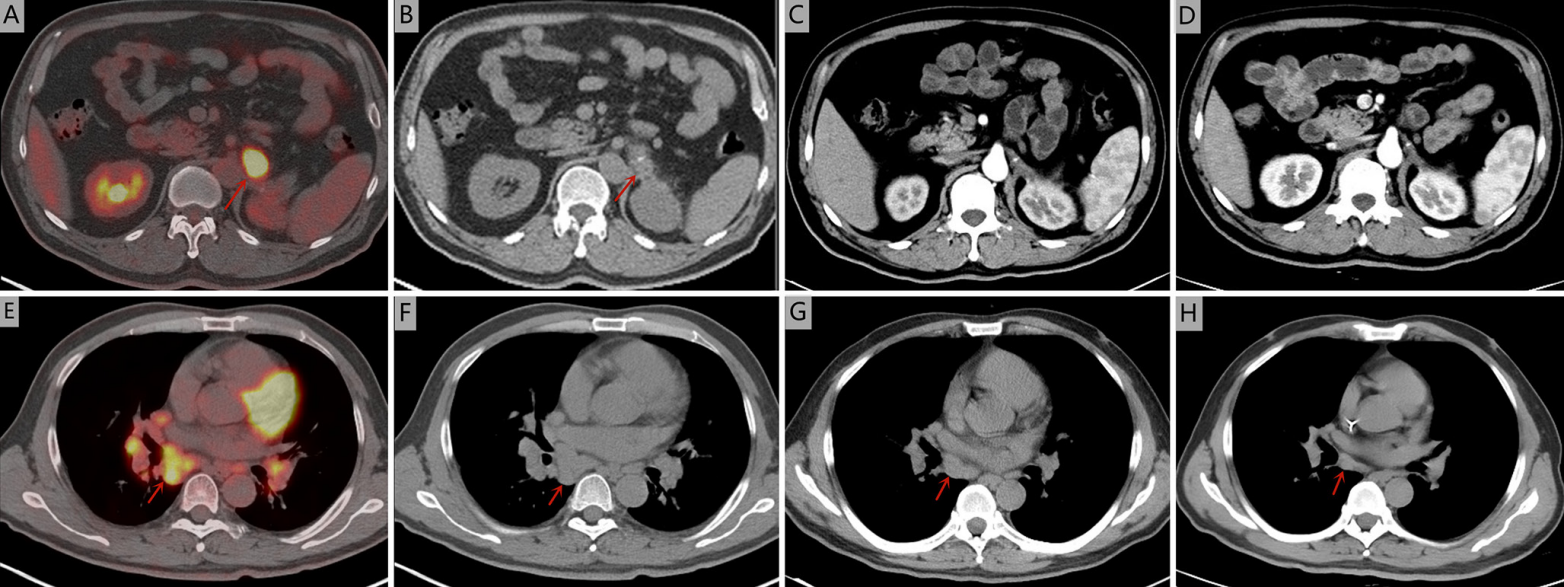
Seven months after operation, positron emission tomography/computed tomography was performed. (A, B) The soft tissue mass in the operative area was accompanied by an abnormal increase of fluorodeoxyglucose metabolism (maximum standardized uptake value, 21.0). (E, F) The larger lymph nodes were located in the 8R area (4.2× 1.9 cm, maximum standardized uptake value, 7.9). Computed tomography during treatment showed that the tumour lesions disappeared in the arterial phase and the mediastinal and mediastinal-hilar lymph nodes were significantly reduced or had disappeared. (C, G) after two cycles of chemotherapy. (D, H) after six cycles of chemotherapy.
